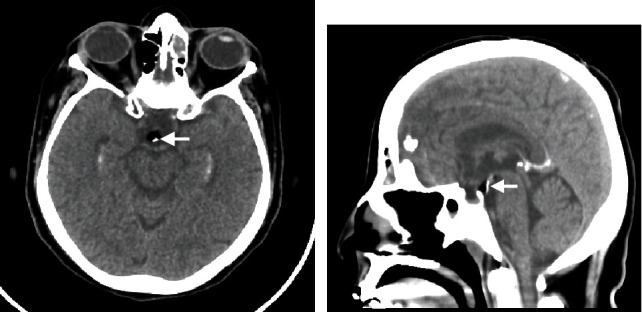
(a) Axial brain CT. (b) Coronal brain CT. (a) and (b) The images demonstrate a small fat-density lesion with punctate calcification, representing a hypothalamic lipoma.
